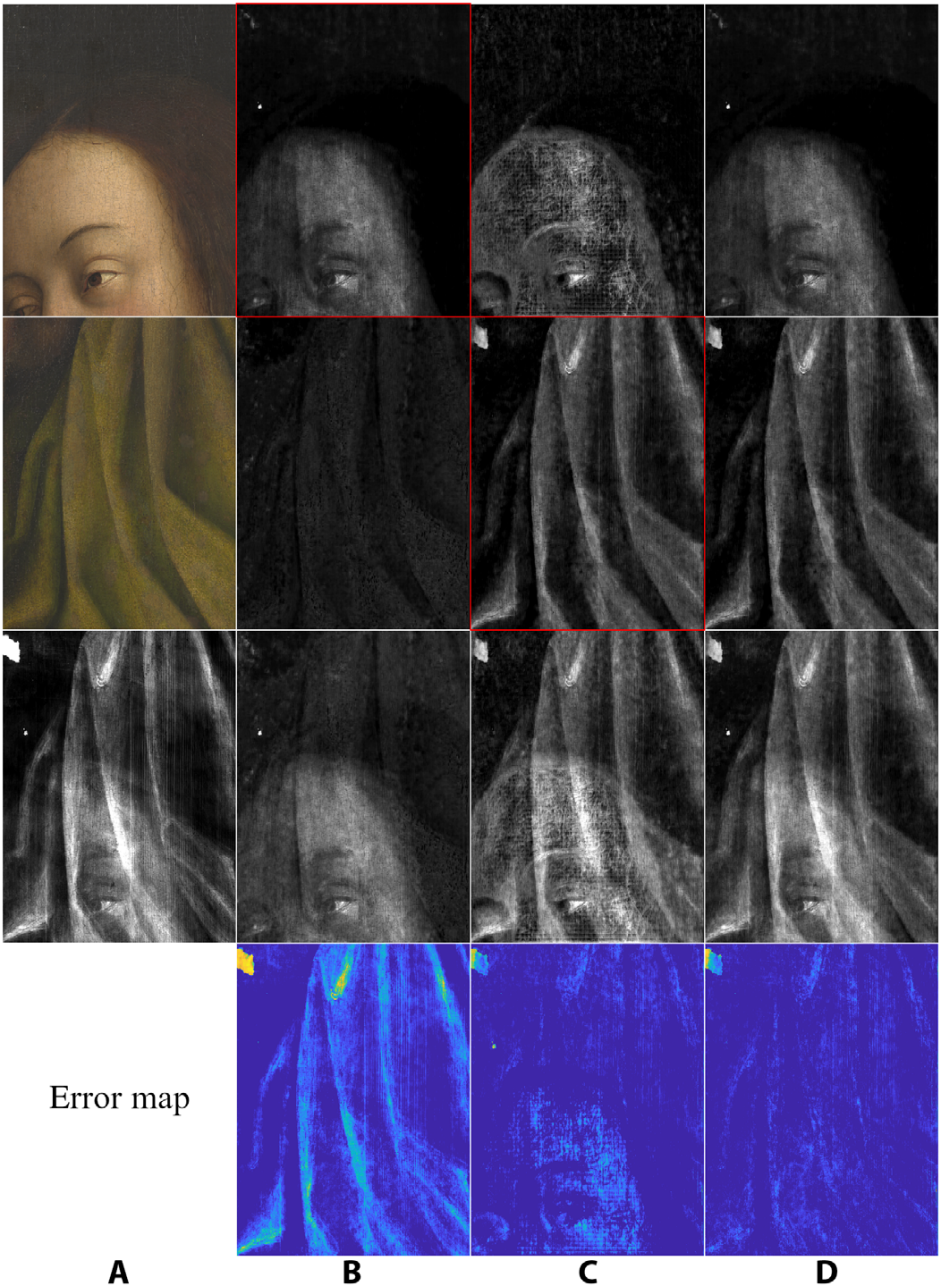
Results of the proposed algorithm applied to a detail from the *Eve* panel. Column (A) input data; (B to D) results from first (B), second (C), and combined (D) approach—the latter takes the best of both first and second approaches. Top row: Interior (*Eve*) (before conservation) and various reconstructed x-ray images. Second row down: exterior (drapery) side RGB input (image mirrored for easier comparison with the x-ray images) and the reconstructed x-ray images. Third row down: Original mixed x-ray input image (left) and mixtures of the reconstructed x-ray images in rows 1 and 2. Bottom row: Visualization of the error map for each approach.
